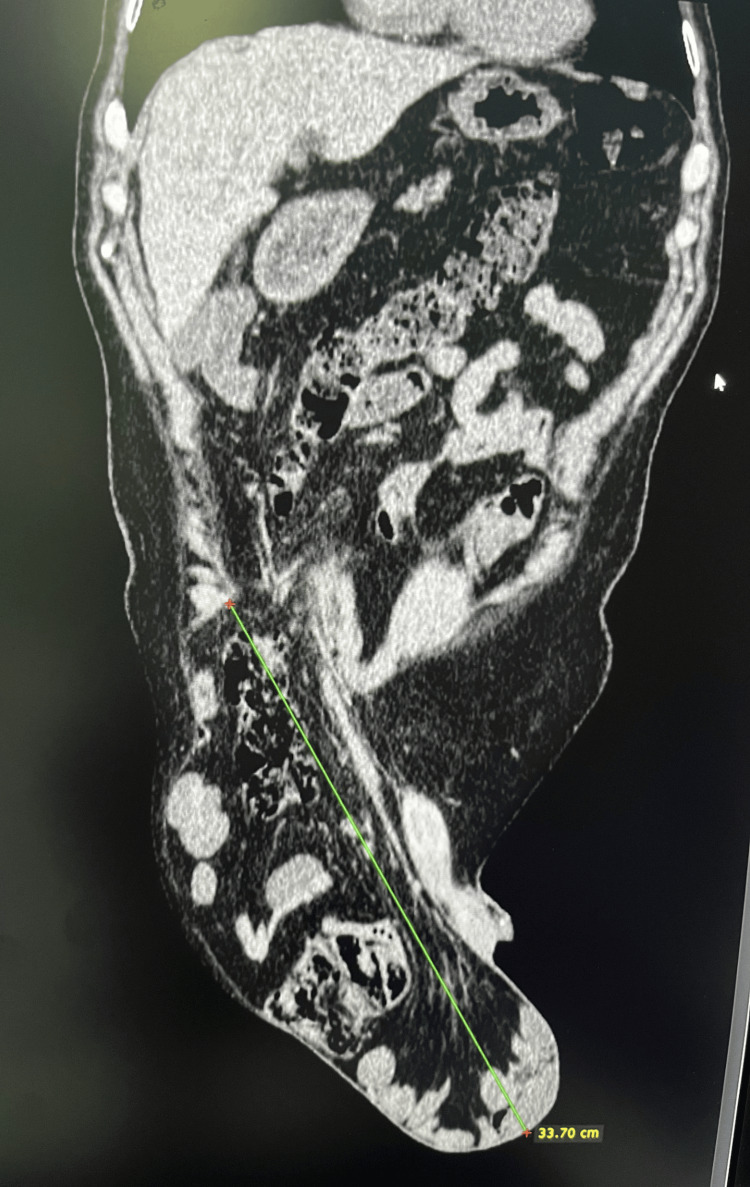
Preoperative CT scan (sagittal view) showing a 33 cm long hernial sac filled with small and large intestines, along with the mesentery
